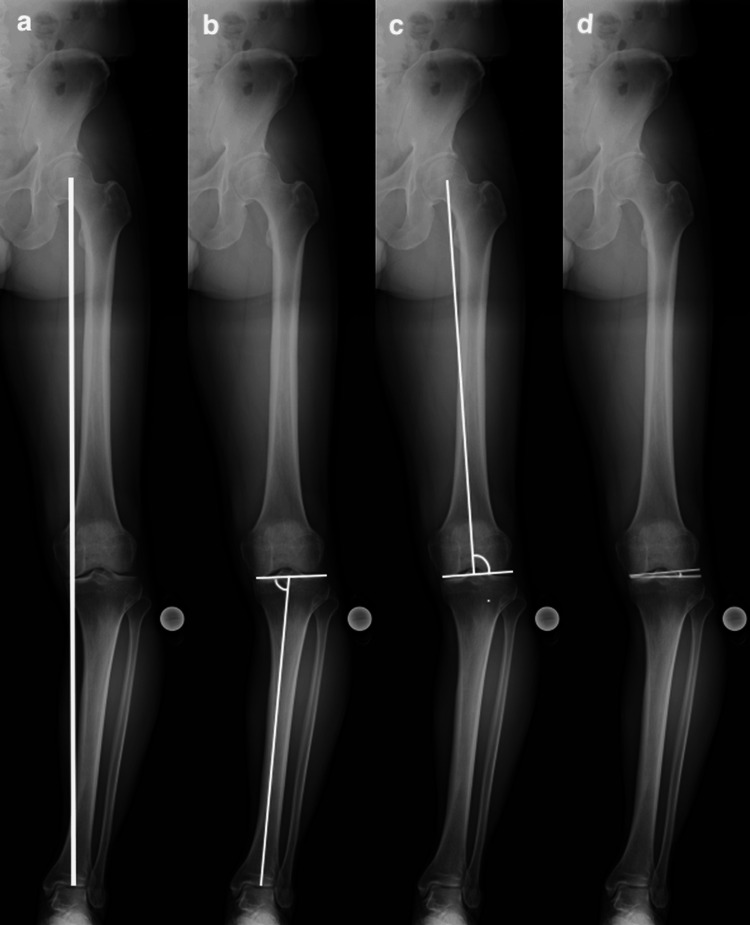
Radiographic parameters on single-leg standing whole-leg radiographs (a) MA: A straight line drawn from the center of the femoral head to the center of the ankle joint. The percentage of the MA is calculated as the ratio of the horizontal distance from the medial edge of the tibial plateau to the intersection of the MA. (b) mMPTA: The medial angle between the tibial MA and the proximal articular surface of the tibia. (c) mLDFA: The lateral angle between the femoral MA and the distal articular surface of the femur. (d) JLCA: The angle between the distal femoral articular surface and the proximal tibial articular surface. MA: Mechanical axis; mMPTA: Mechanical medial proximal tibial angle; mLDFA: Mechanical lateral distal femoral angle; JLCA: Joint line convergence angle Image credit: Author Tetsuro Shimada
